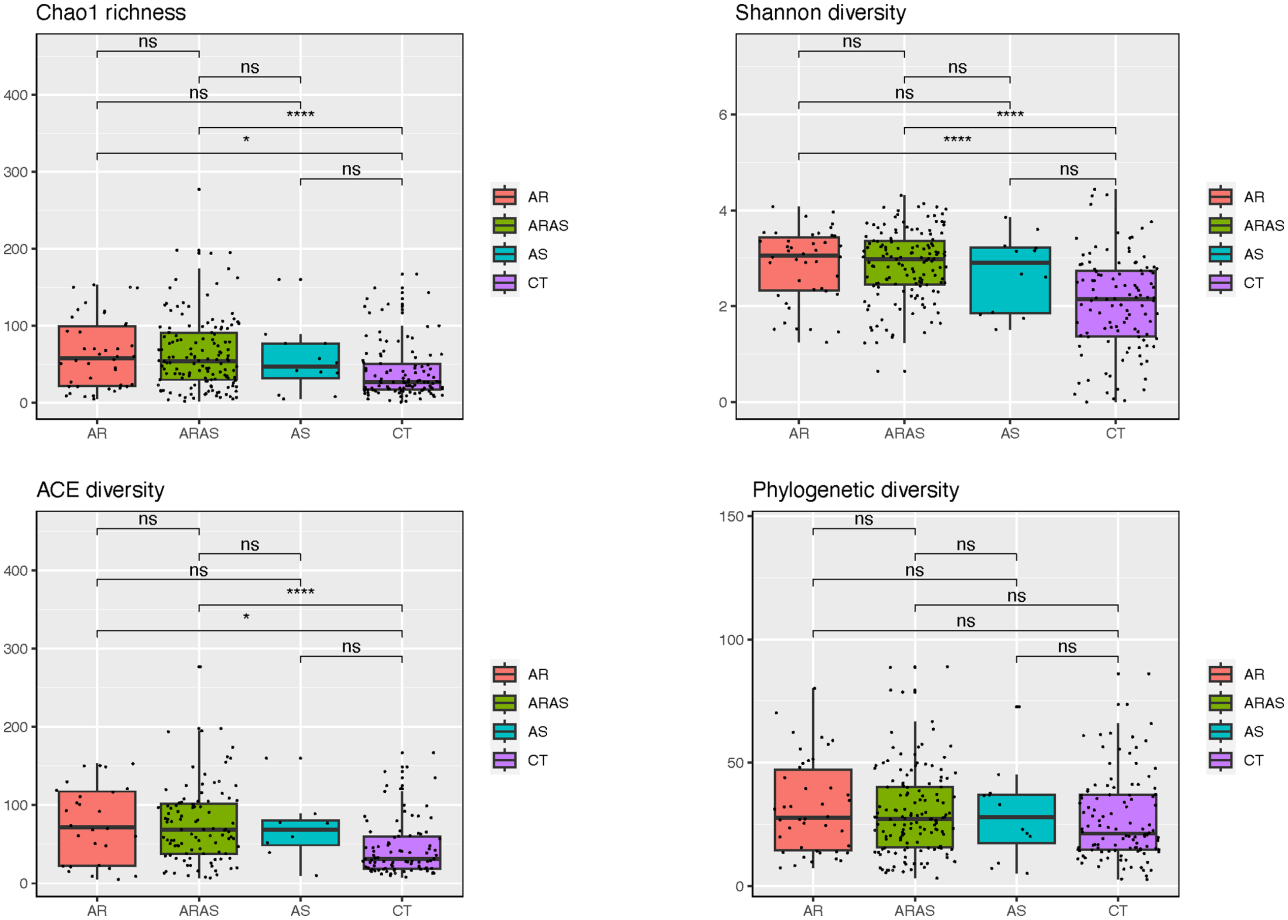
Bar plots of mean relative proportions of the top fungal genera in the nasal cavity of participants with allergic rhinitis (AR), AR with comorbid asthma (ARAS), asthma (AS), and healthy controls (CT).
